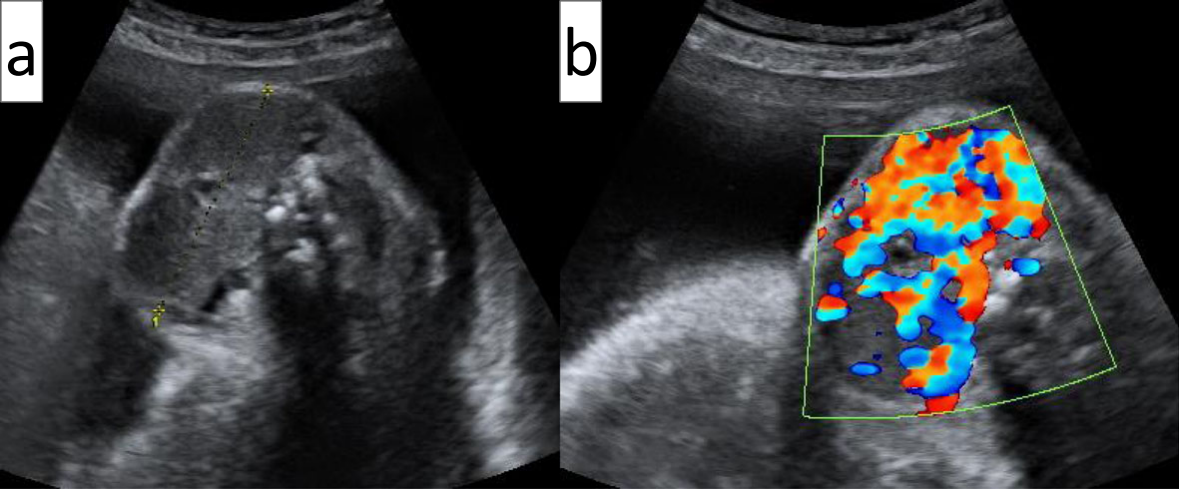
Fetal ultrasound images. Both images are taken at 34 weeks and 4 days of gestation. a: An image of the transverse section of the fetal neck. The transverse diameter and circumference of the fetal thyroid were 58.4 and 178 mm, respectively. b: An image of the transverse section of the fetal neck obtained with an ultrasonic Doppler blood flowmeter. The blood flow was diffusely increased
